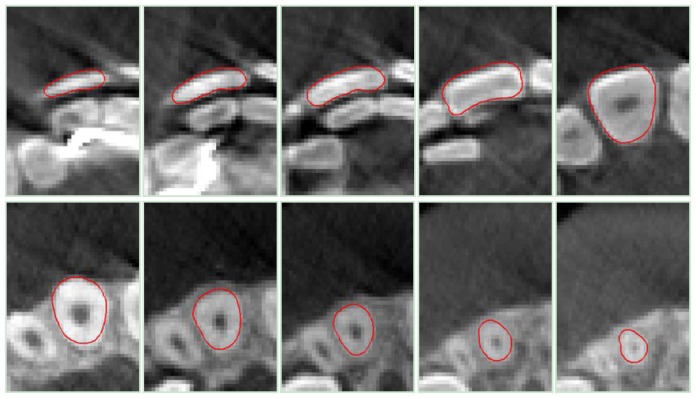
Results of the DRLSE method for *tooth 11*, obtained by processing some CBCT slice images.
